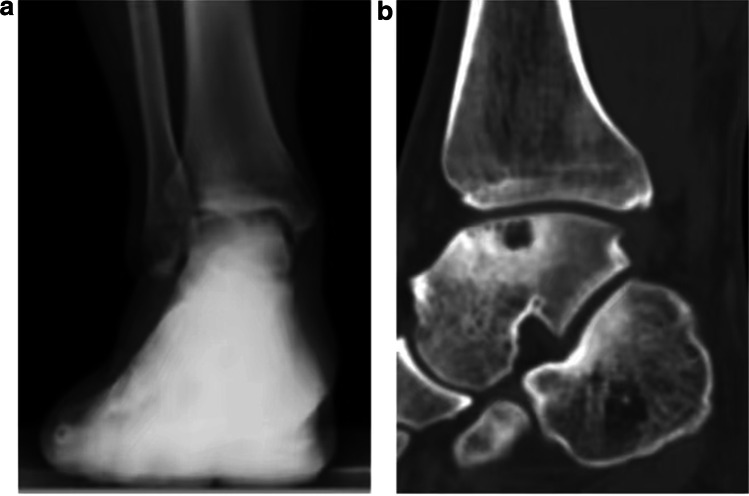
Pre-operative X-ray and CT. a Pre-operative X-ray showing that the local bone density of the medial talus was reduced. b Pre-operative CT showing that the local sac of the talus fornix becomes obvious and a cavity was formed
